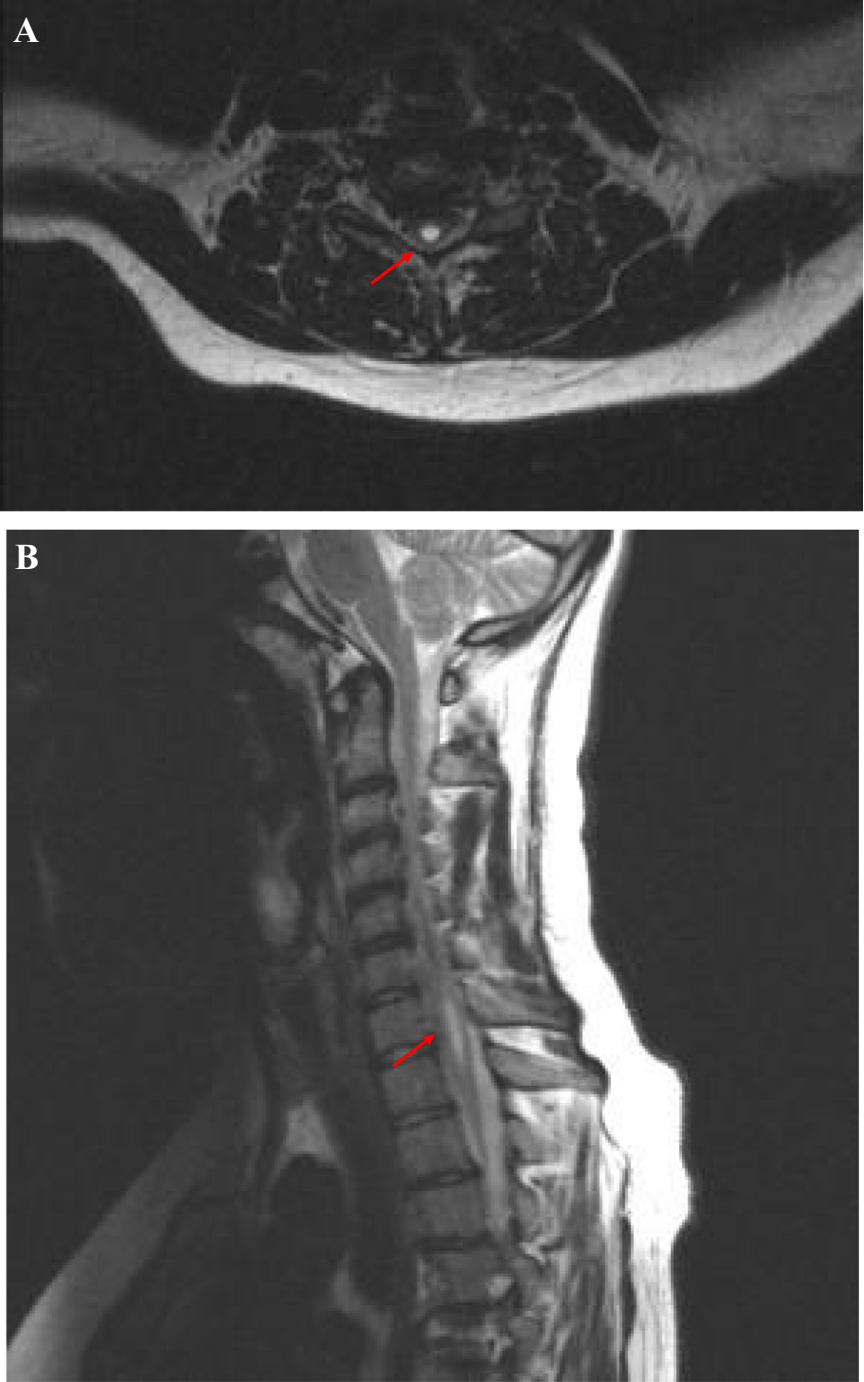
MRI of the spine. A: axial T2-weighed MRI of the cervical spine showing syrinx formation at the level of 6th cervical vertebra (arrow). B: sagittal T2-weghed MRI of the spine demonstrating type 1 Chiari malformation and associated cervico-dorsal (C6-T1) syringomyelia.
